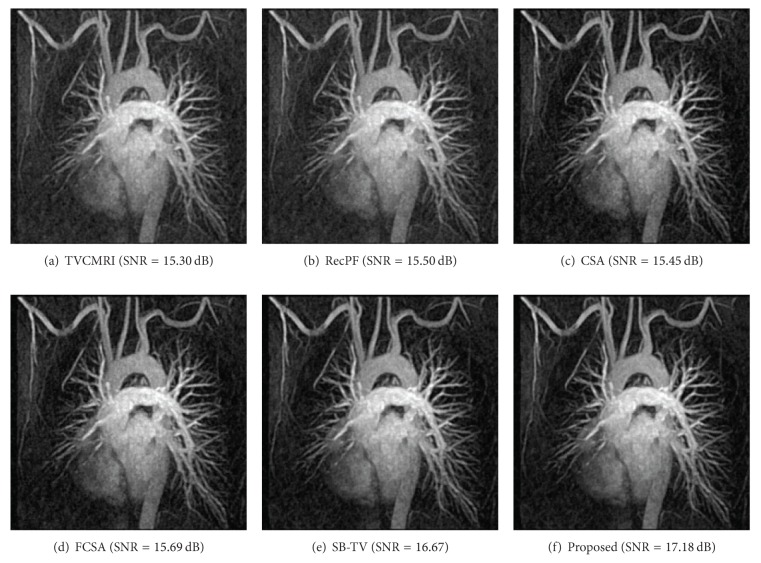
Chest MR image reconstruction from 20% samples (Rician noise case).
